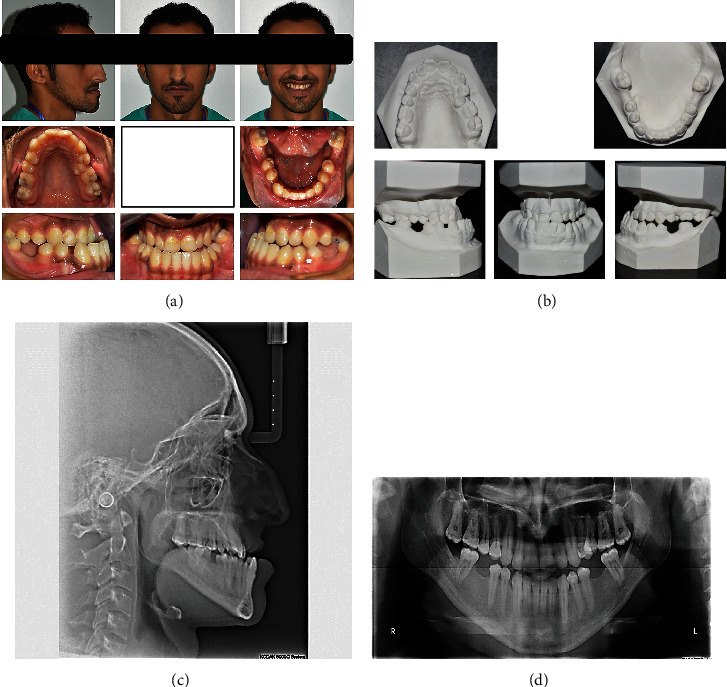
Pretreatment extraoral and intraoral photographs, study models cephalometric radiograph, and panoramic radiographs of the patient: (a) extraoral and intraoral photographs; (b) study models; (c) cephalometric radiograph; (d) panoramic radiograph.
